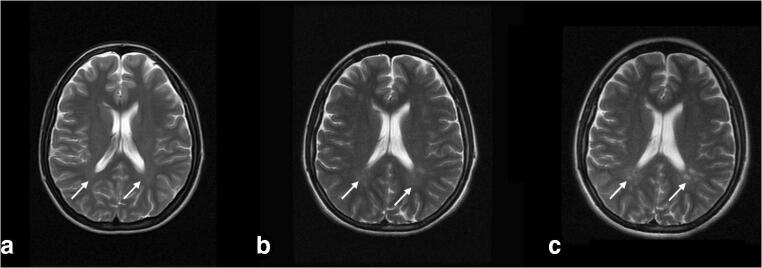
Brain IRM FLAIR sequence year 2007 (a), 2011 (b), and 2012 (c). Bilateral progressive periventricular hyperintensities around the posterior horns of the lateral ventricles (white arrows)
